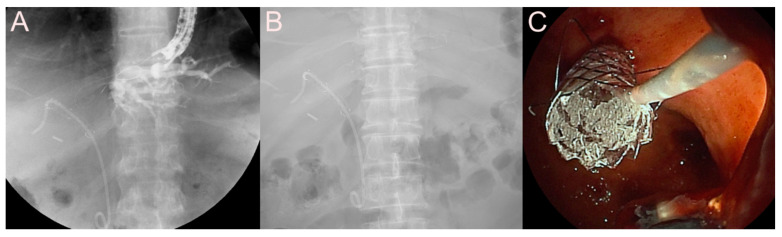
Ultrasound-guided hepatico-gastrostomy using the dedicated partially covered self-expandable metal stent (PCSEMS) (hybrid metal stents; Standard Sci Tech Inc., Seoul, Korea) for the obstruction of the left intrahepatic duct (IHD). (A) Fluoroscopic image showing a 19-gauge needle puncture into the left dilated IHD. (B) Fluoroscopic image showing the dedicated PCSEMS placed between the stomach and the left IHD. (C) Endoscopic image showing the drainage of pus-like materials through the dedicated PCSEMS.
